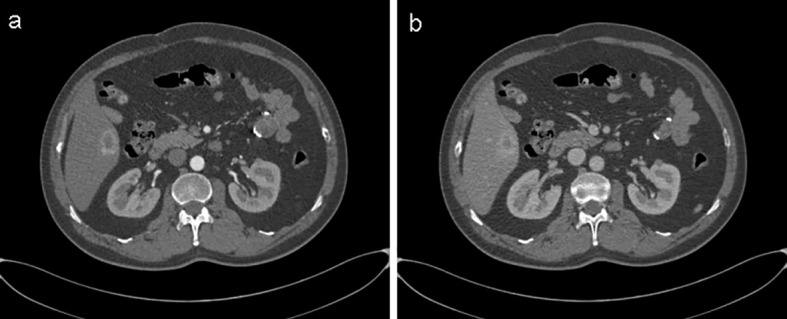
Abdominal contrast-enhanced CT revealed a hypodense hepatic nodule in the right posterior inferior lobe, measuring approximately 2.9 × 1.9 cm. (a) Arterial-phase imaging exhibited marked heterogeneous rim-like enhancement, while (b) portal venous-phase imaging demonstrated persistent enhancement, with a central area of comparatively diminished attenuation.
